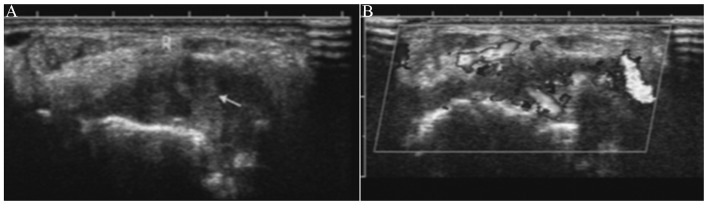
(A) Synovial thickness of the second metacarpophalangeal joint (arrow represents hyperplasia of the synovium). (B) Level 3 synovitis of the second metacarpophalangeal joint in color Doppler.
